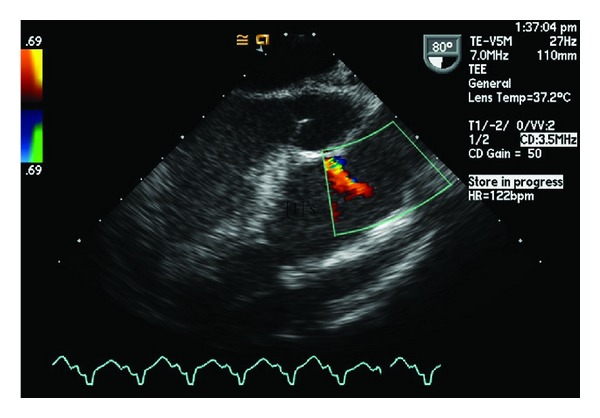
Transesophageal echo demonstrating the pulmonary insufficiency was believed to be secondary to the position of the outflow cannula of the right ventricular assist device.
